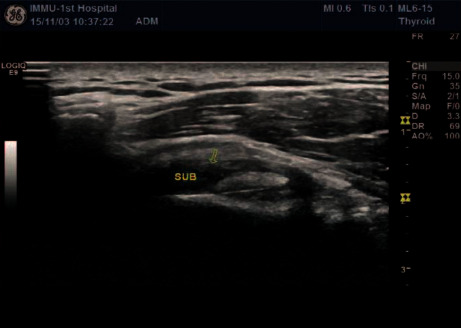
Two-dimensional ultrasound subscapular tendon injury.
